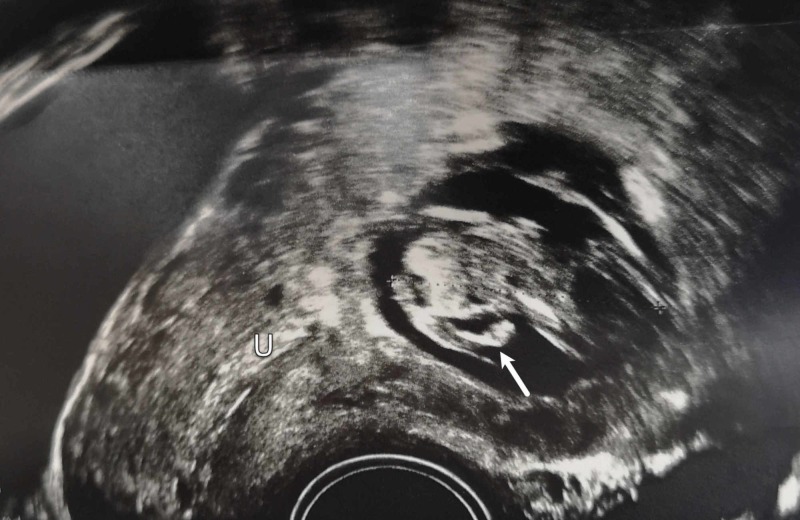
Transvaginal Ultrasound Scan Transvaginal ultrasound image showing the presence of gestational sac with fetal pole (arrow) located in the anterior part of the lower uterine segment with empty uterine cavity (U).
